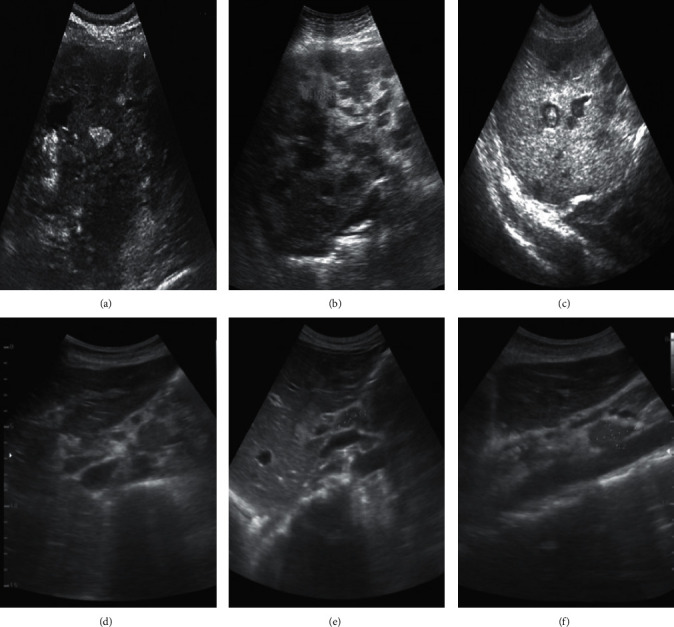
Ultrasound images of breast cancer axillary lymph node metastasis (note: (a–c) were left axillary lymph nodes; (d–f) were right axillary lymph nodes).
